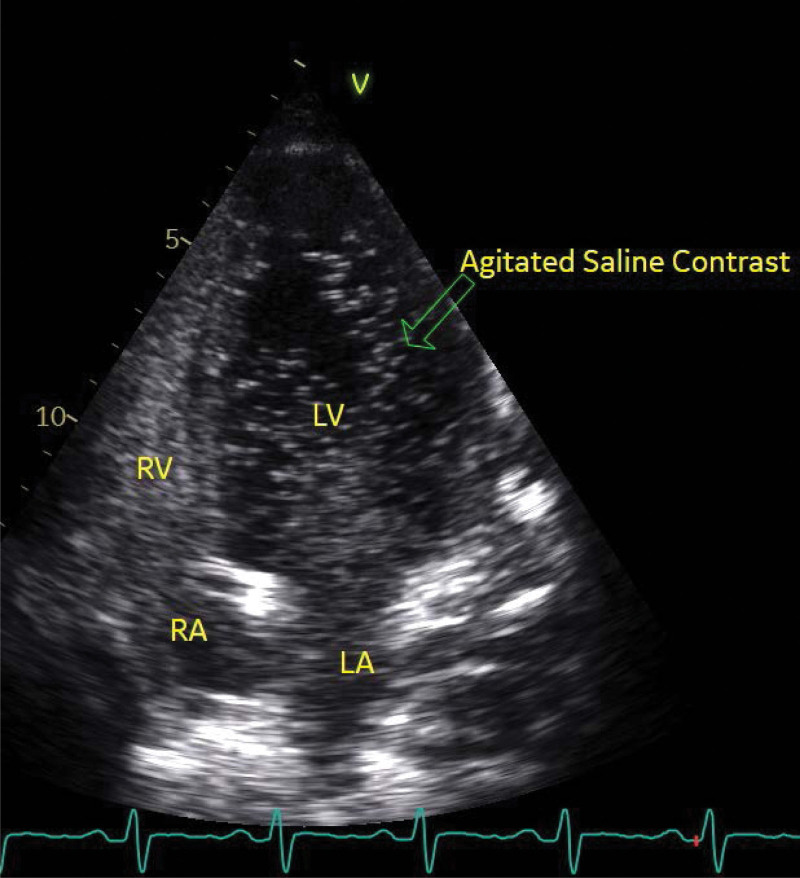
Four-chamber echocardiography view, which shows evidence of early bubble contrast passage through interatrial septum indicating the presence of patent foramen oval. LA = left atrium, LV = left ventricular, RA = right atrium, RV = right ventricular.
